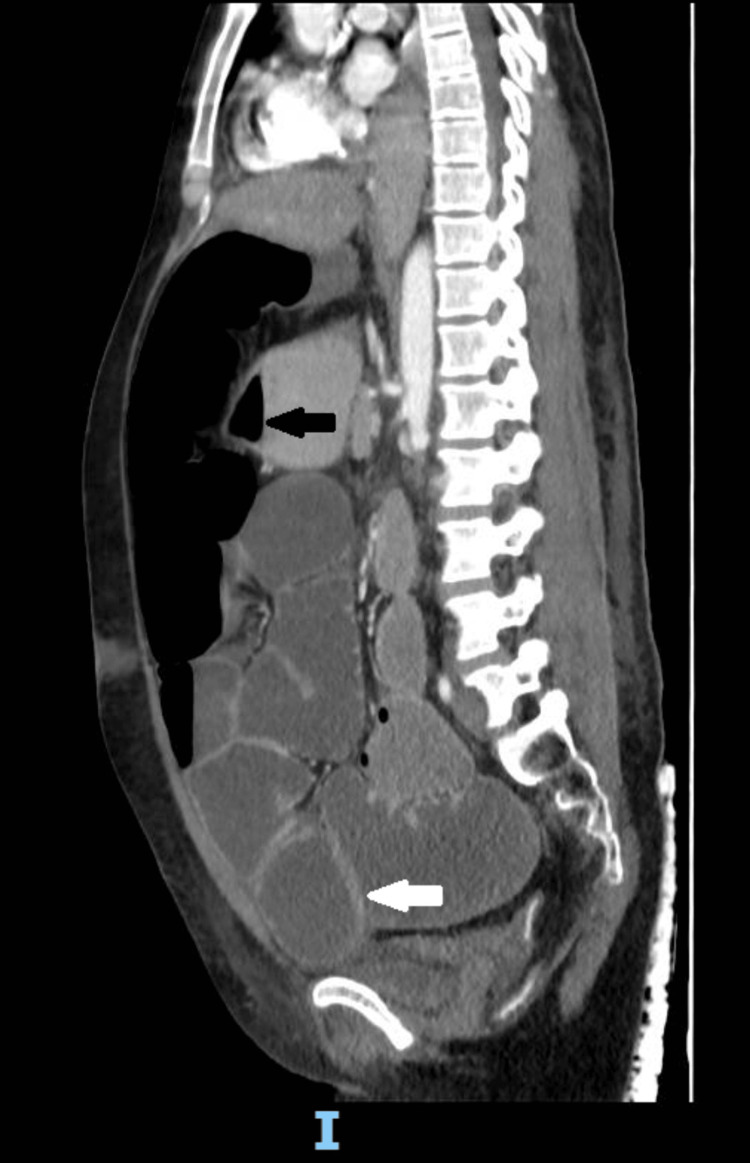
CECT of the abdomen (sagittal section) showing air-fluid levels (black arrows) and bowel wall thickening (white arrow) CECT, contrast-enhanced computed tomography
